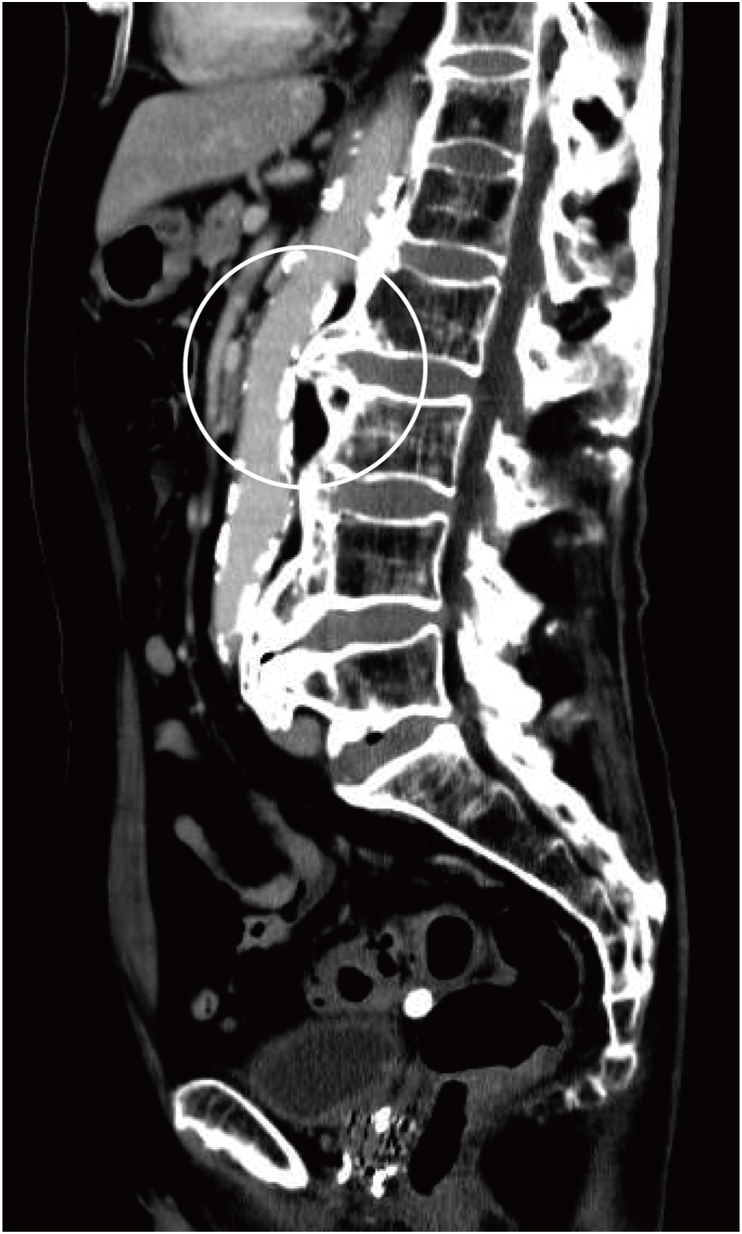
Sagittal section of CT, which was taken 2 years ago. A sharp osteophyte (white circle) in the lumbar spine (L 2/3), which was in touch with the abdominal aorta.
